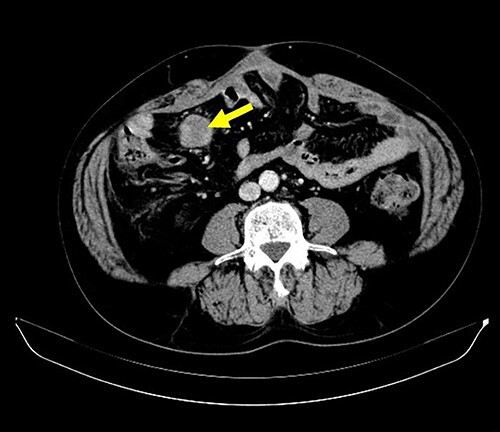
CT finding. Follow-up CT after gastrectomy for gastric cancer revealed a mesenteric tumor. There were no other malignant findings.
